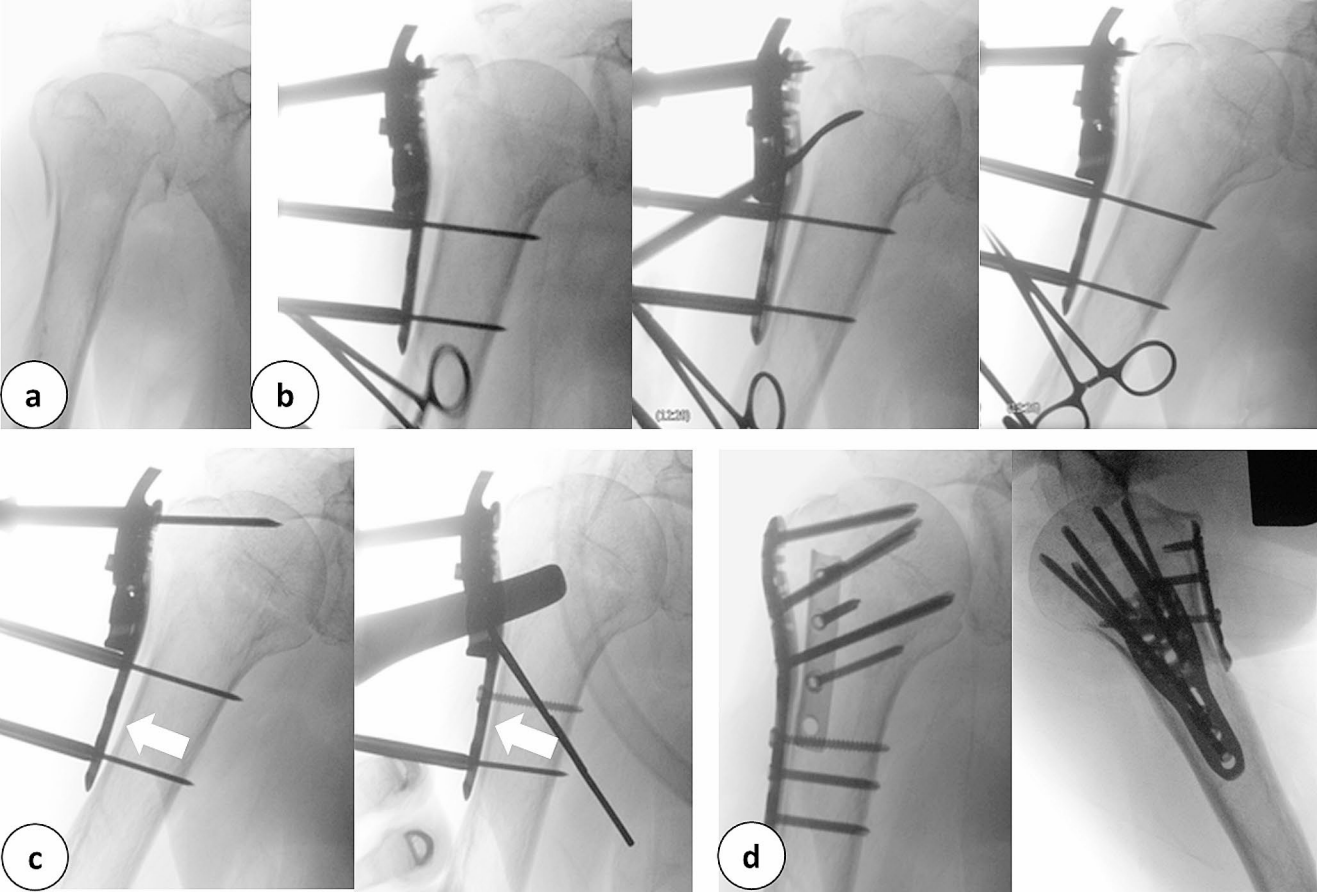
a: Intraoperative anterior-posterior radiograph of a multifragmentary proximal humerus fracture. b: Application of the stable-angle plate osteosynthesis and elevation of the dislocated humeral head with the raspatory. c: Fixation of the plate in the area of the humeral head and shaft with Kirschner wires and reduction of the axis by a cortical screw over the plate (white arrows). d: Final radiographic control with double plate osteosynthesis in two planes
